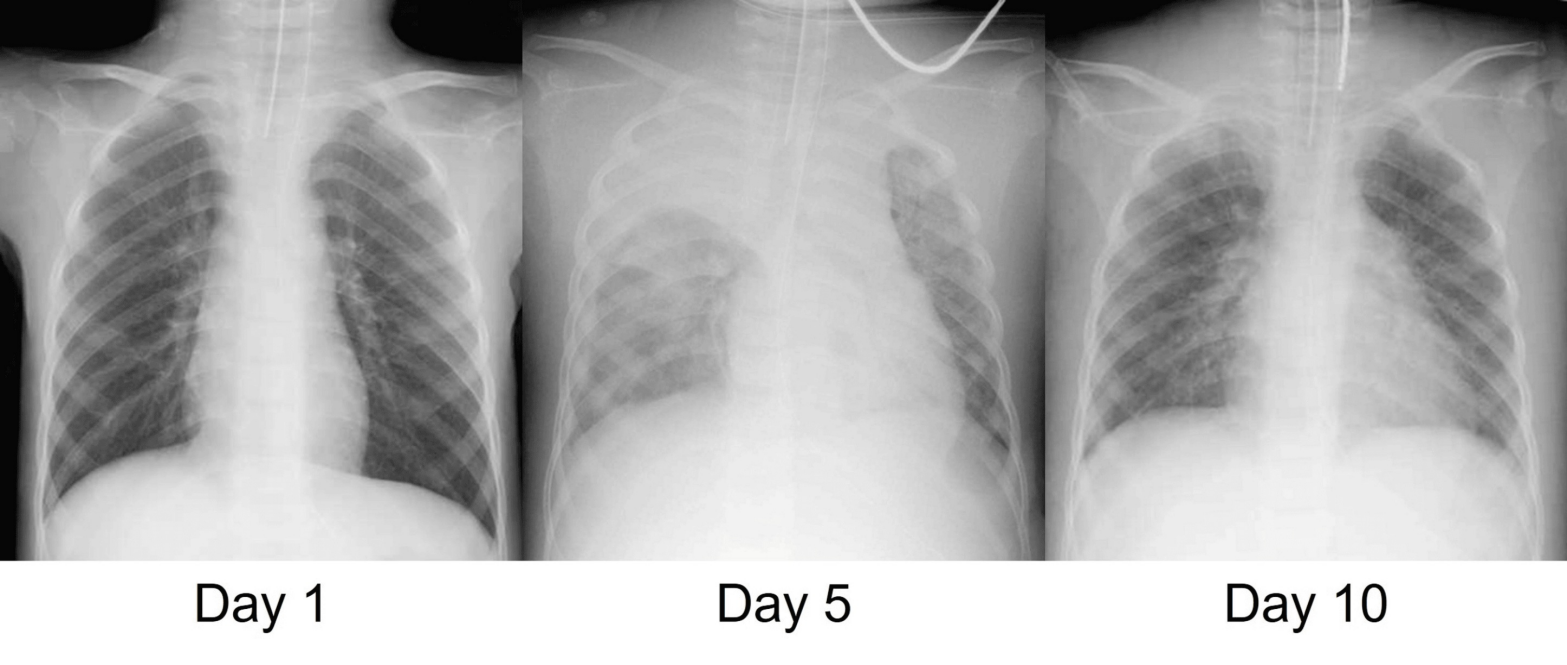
Chest X-ray on Days 1, 5, and 10. Day 5 highlights severe pulmonary edema and atelectasis, which compromised respiratory function and necessitated the initiation of ECMO. Day 10 demonstrates significant resolution of these findings following the initiation of integrated CRRT, which allowed for precise fluid removal, resulting in the improvement of the patient’s respiratory status. ECMO, extracorporeal membrane oxygenation; CRRT, continuous renal replacement therapy
